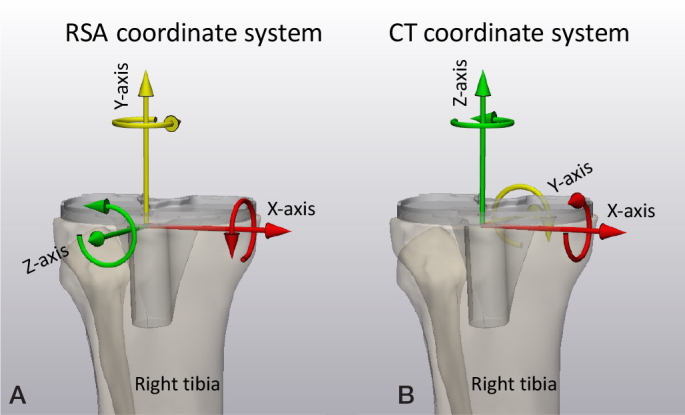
(A) The RSA migration coordinate system for a right-sided tibia with X-axis pointing medially, Y-axis pointing superiorly, and Z-axis pointing anteriorly. (B) The CT migration coordinate system for right-sided tibia with X-axis pointing medially, Y-axis pointing posteriorly, and Z-axis pointing superiorly.
